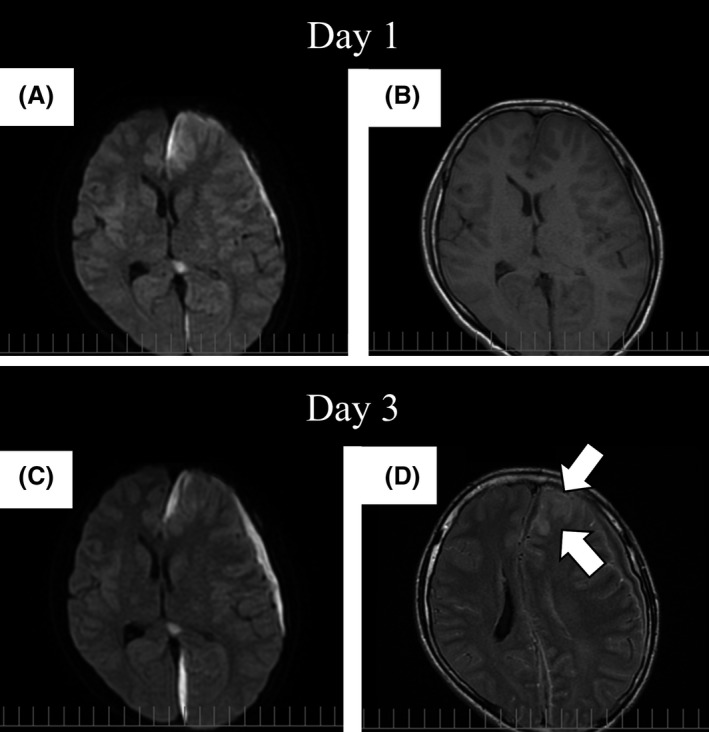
Magnetic resonance imaging studies performed on the day of admission and day 3. (A and C) Despite intravenous antibiotic therapy, enlargement of the subdural abscess is observed in diffusion‐weighted image sequences. (B and D) A high‐intensity area appeared at the left frontal lobe (arrows) in a fluid‐attenuated inversion recovery sequence taken on day 3, and spread of infection was suspected. The midline shift also worsened.
